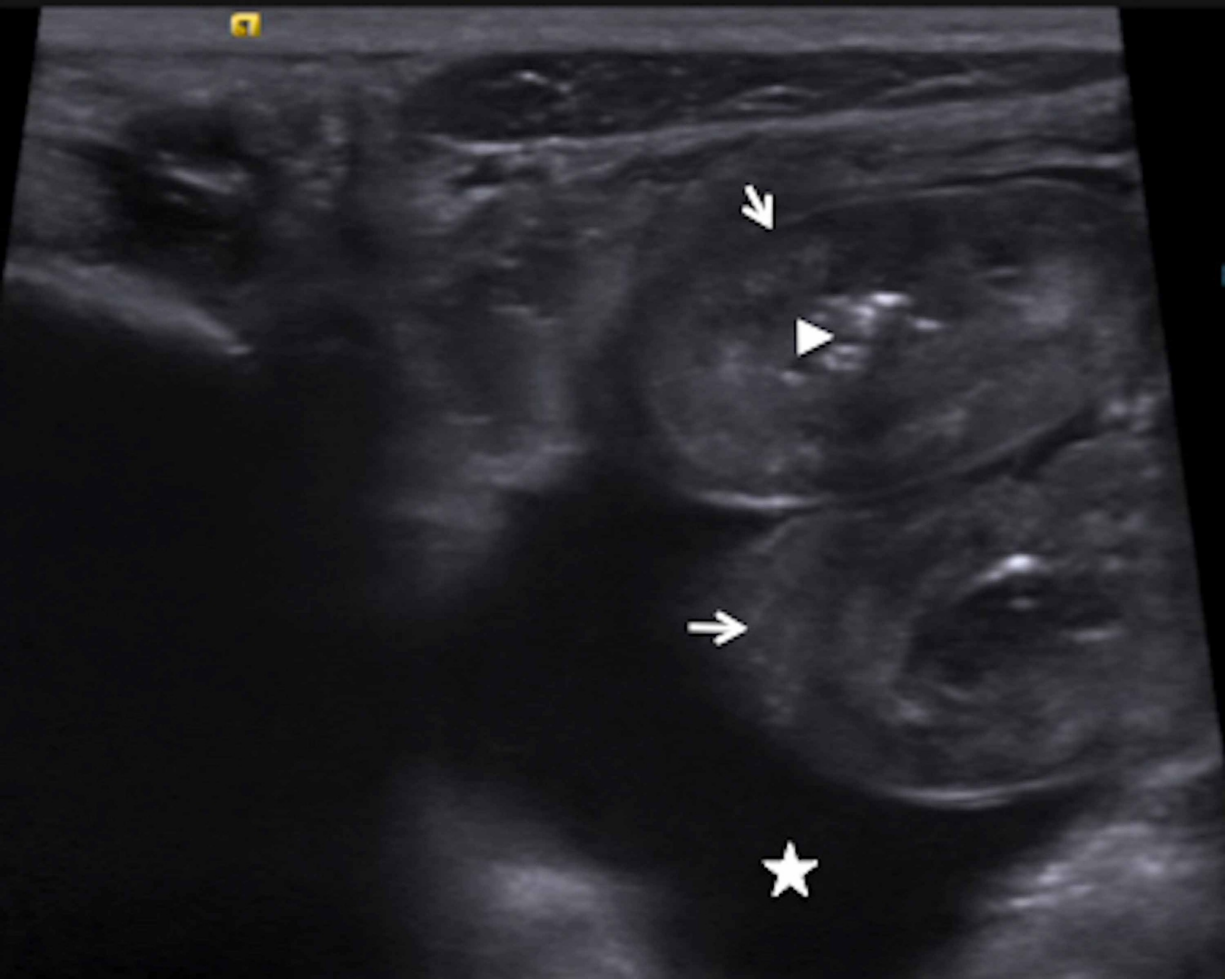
Repeat Abdominal Ultrasound Image of the lower abdomen midline. Again several loops of small bowel with concentric bowel thickening (arrows) and associated simple-appearing ascites (star) are seen. Some bowel loops are fluid-filled, debris-filled and/or gas-filled (arrowhead demonstrates gas in the lumen). Real-time scanning showed no bowel intussusception or intraperitoneal fluid collection.
